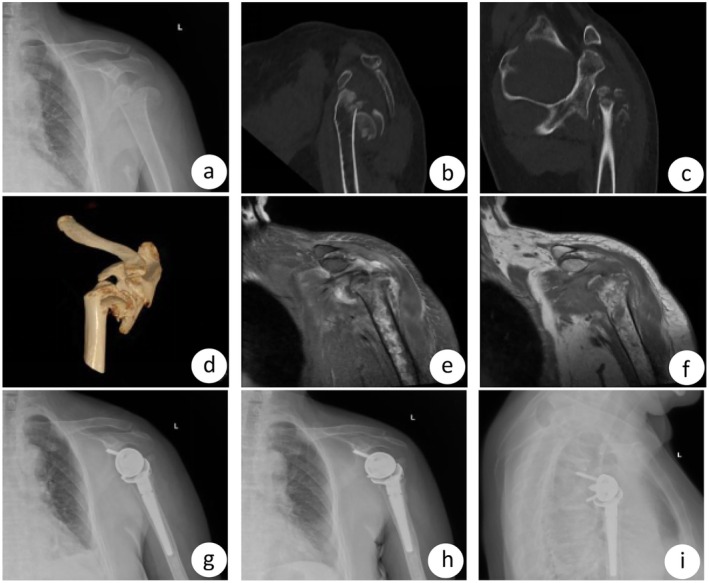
(a) Preoperative radiograph showing a comminuted fracture of the proximal humerus with bone destruction. (b–d) Preoperative CT scans showing a comminuted fracture of the proximal humerus with multiple abnormal signals in the medullary cavity. (e, f) Preoperative MRI revealing a comminuted fracture of the proximal humerus with rotator cuff injury and multifocal abnormal signals in the medullary cavity, suggestive of a marrow‐based lesion. (g) Postoperative radiograph showing satisfactory prosthesis positioning. (h, i) Radiographs at 15 months postoperatively showing the prosthesis remains well positioned with no significant changes compared to (g).
